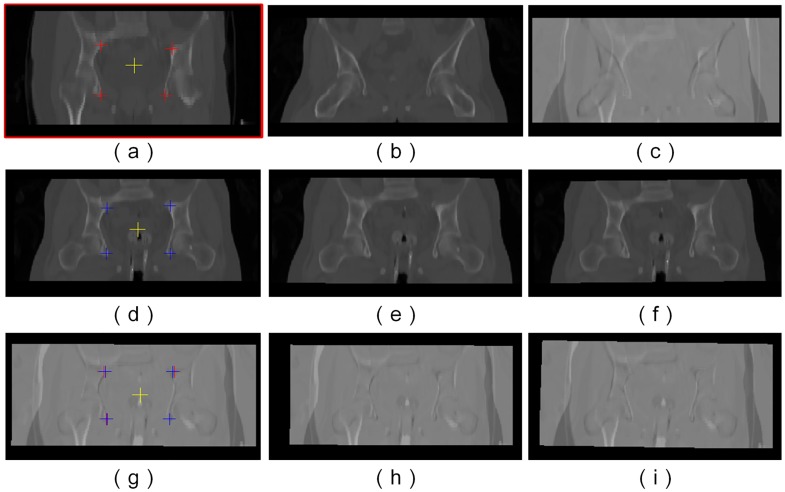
Coarse registration between EBRT and HDR-BT CT images for better initialization. (a) EBRT images used as a reference. (The four marked points inside the image were used for point set rigid registration.) (b) HDR-BT image before initialization. (c) Difference images between EBRT and HDR-BT before initialization. (d) HDR-BT image with four marked points used for point set rigid registration. (e) HDR-BT image after intensity-based rigid, and affine (f) registration. The corresponding difference images between EBRT and HDR-BT after point set rigid, intensity-based rigid, and affine registration are shown in (g)–(i).
